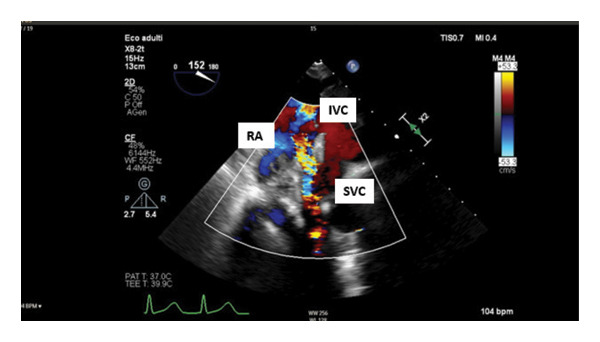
Deep transgastric color Doppler flow velocity in the setting of acute vena cava stenosis. RA: right atrium; IVC: inferior vena cava; SVC: superior vena cava.
